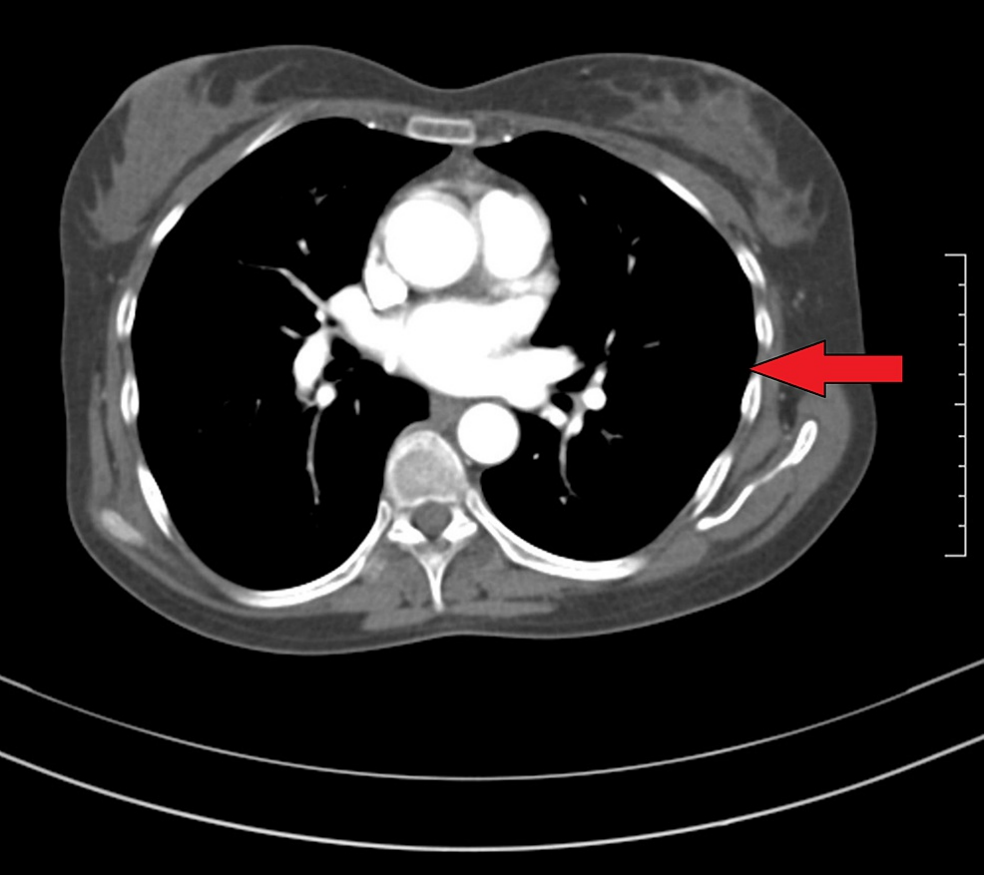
CT chest showing resolved left-sided pleural effusion after six months.
